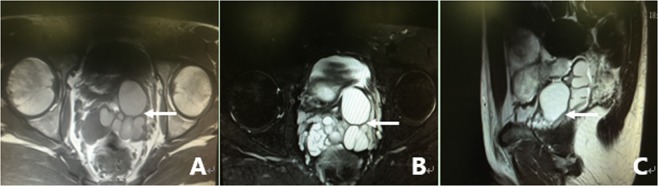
MRI image of SVC. (A) This image shows dilatation or enlargement in the left SV and presentation as a cystic structure with multiple cavities, with middle to high signal intensity in the left SV on T1WI. (B) This cyst presented as high signal intensity on T2WI, which is similar to that of the right SV. (C) The left SV presented as an obvious dilated cystic structure on the sagittal plane. This pattern suggests that the patient had a left SVC. There is also obvious dilation in the right SV.
